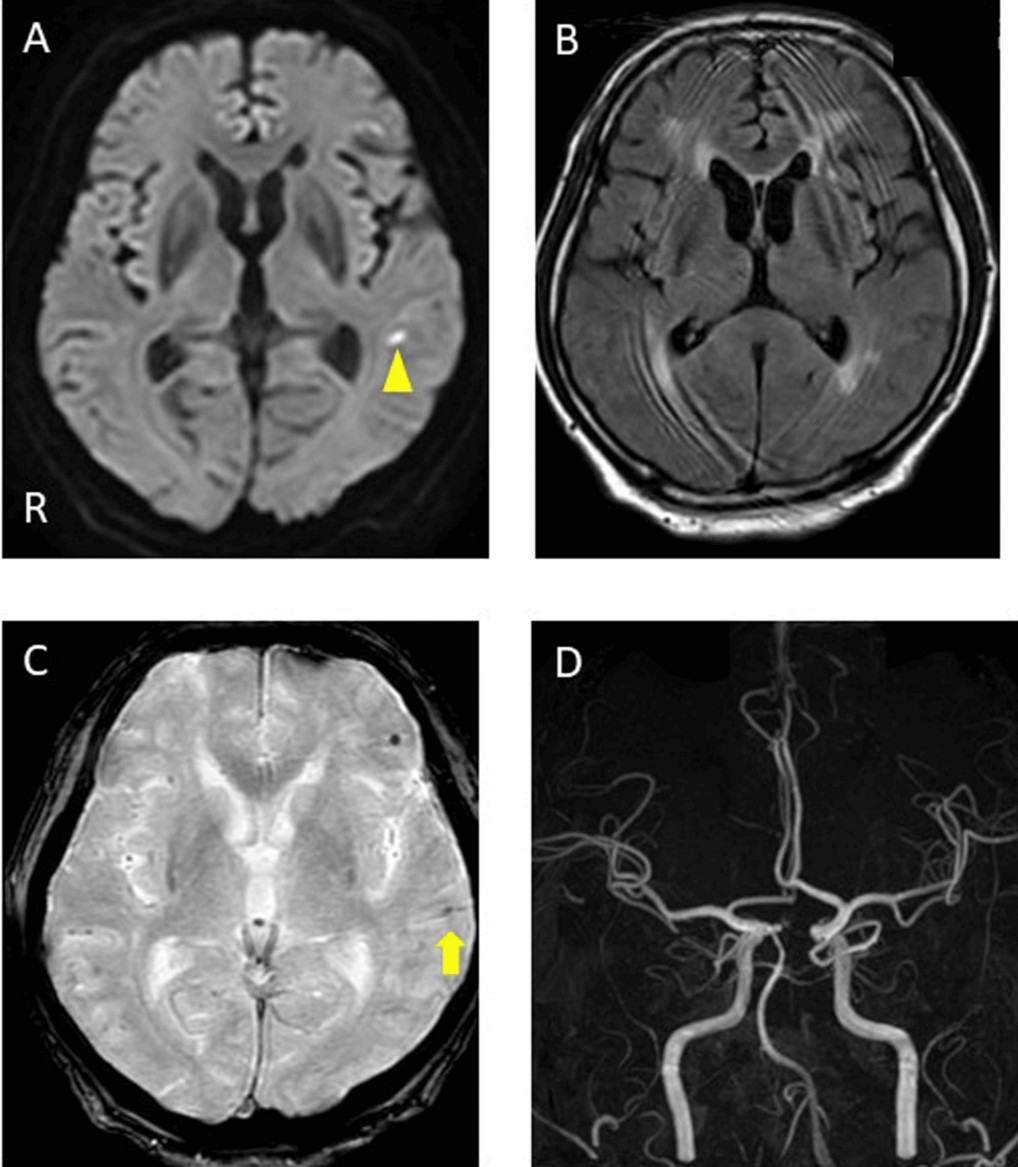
Brain magnetic resonance imaging (MRI) and magnetic resonance angiography (MRA) on day 21 A: Diffusion-weighted imaging (DWI) (3T, b =1000 s/mm², horizontal slice). A small high-signal area was observed in the left temporal lobe (yellow triangle). B: Fluid-attenuated inversion recovery image (FLAIR) showed white matter lesions on both sides. C: T2* imaging demonstrated new microbleeds in the left sulcus (yellow triangle) and cortical superficial siderosis in the left temporal lobe (yellow arrow). D: MRA showed no stenosis or occlusion of the main arteries. R: right
